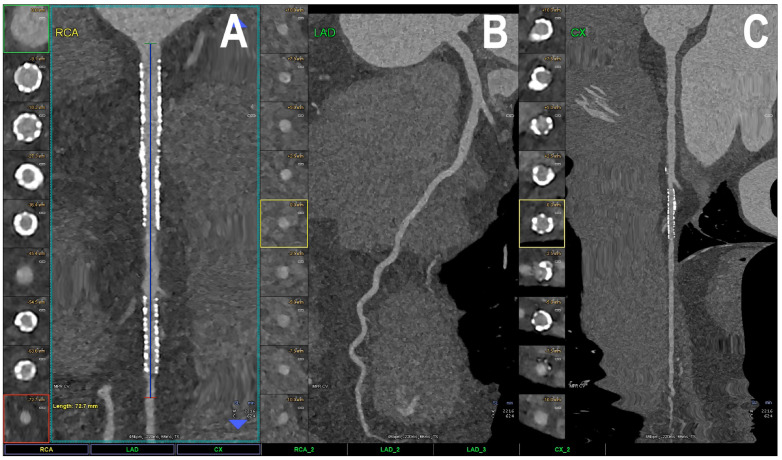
Cardiac PCCT example of a complete coronary tree with multiple stents. The figure shows the complete coronary tree of a patient with multiple stents at follow-up (A–C). There are two stents at the level of the proximal and middle right coronary artery (A) and one stent on the marginal branch of the left circumflex coronary artery (C); the left anterior descending (LAD; (B)) the coronary artery is normal without any detectable atherosclerotic disease. All stents are perfectly visualized in their inner struts and also in their inner lumen, which is not normal for cardiac CT without photon counting technology.
